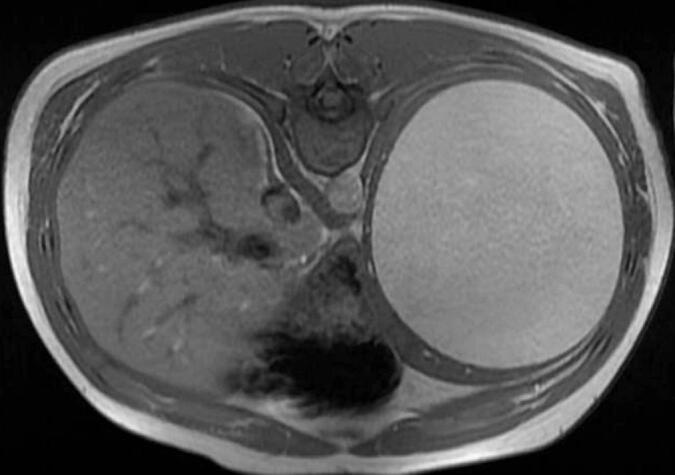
Magnetic Resonance Imaging (MRI) of the abdomen showing a unilocular cystic lesion within the spleen measuring 14.8 × 13.5 × 14.1 cm with high T1 signal intensity.
